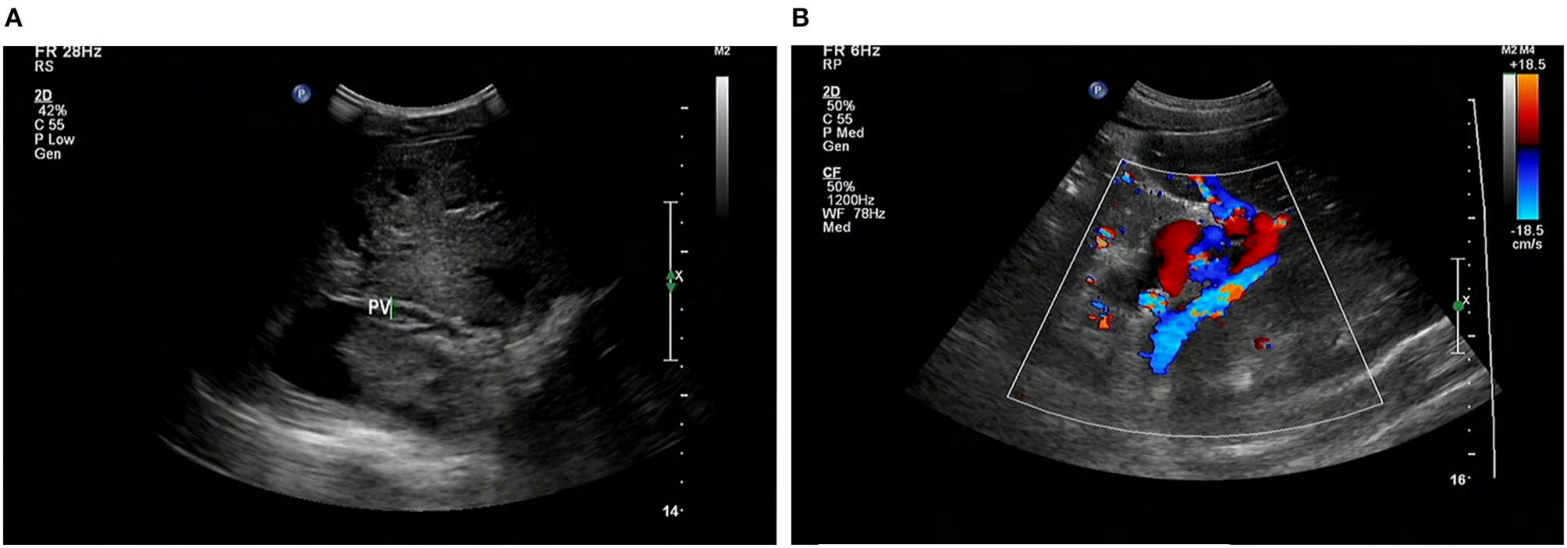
Abdominal US shows that the intrahepatic PV system is absent (arrows) (A), and the GDV is tortuous and dilated (B). US, ultrasonography; PV, portal vein; GDV, gastric fundus vein; STO: stomach.
